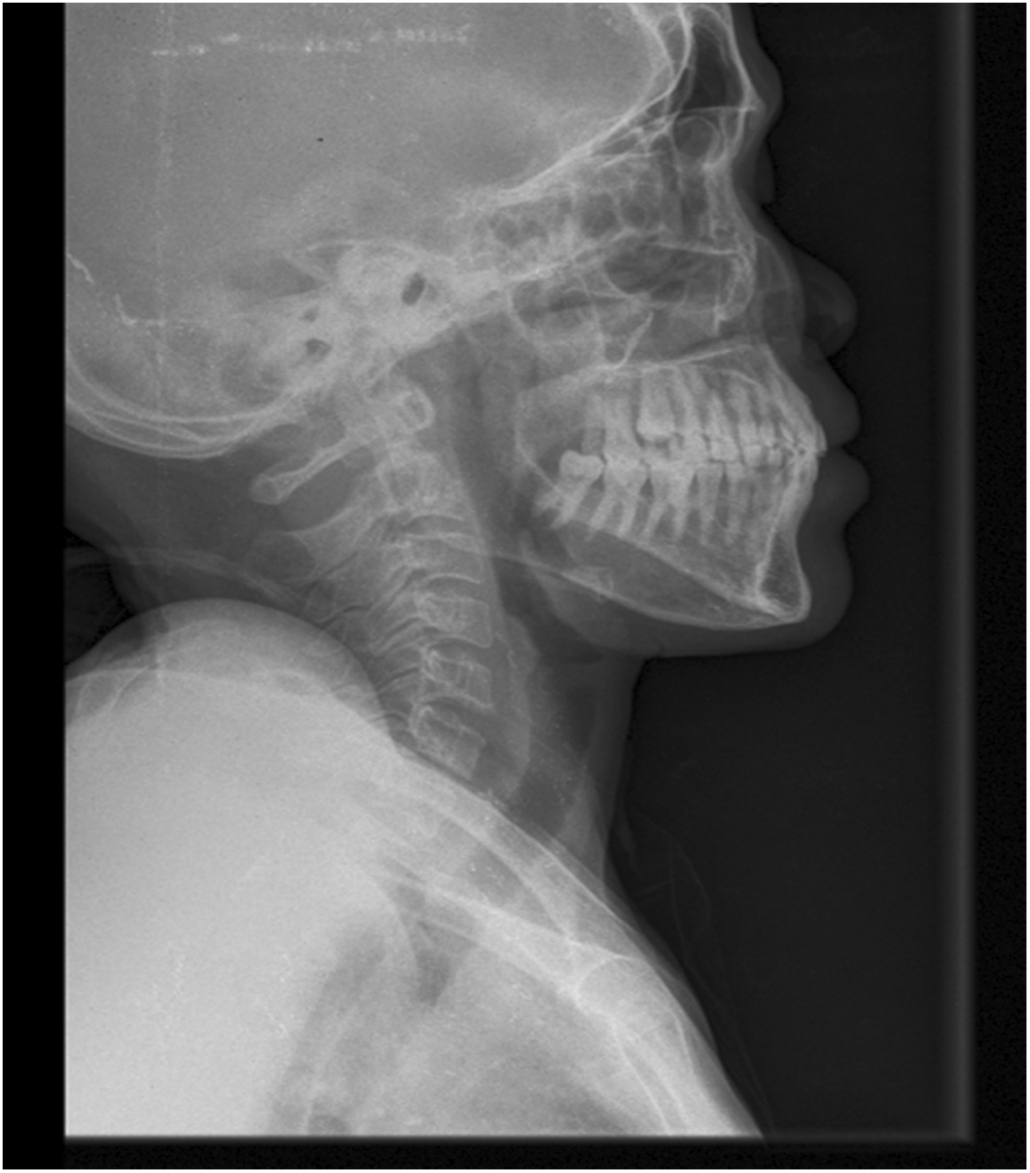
Skull radiograph reveals areas of radiolucency.
